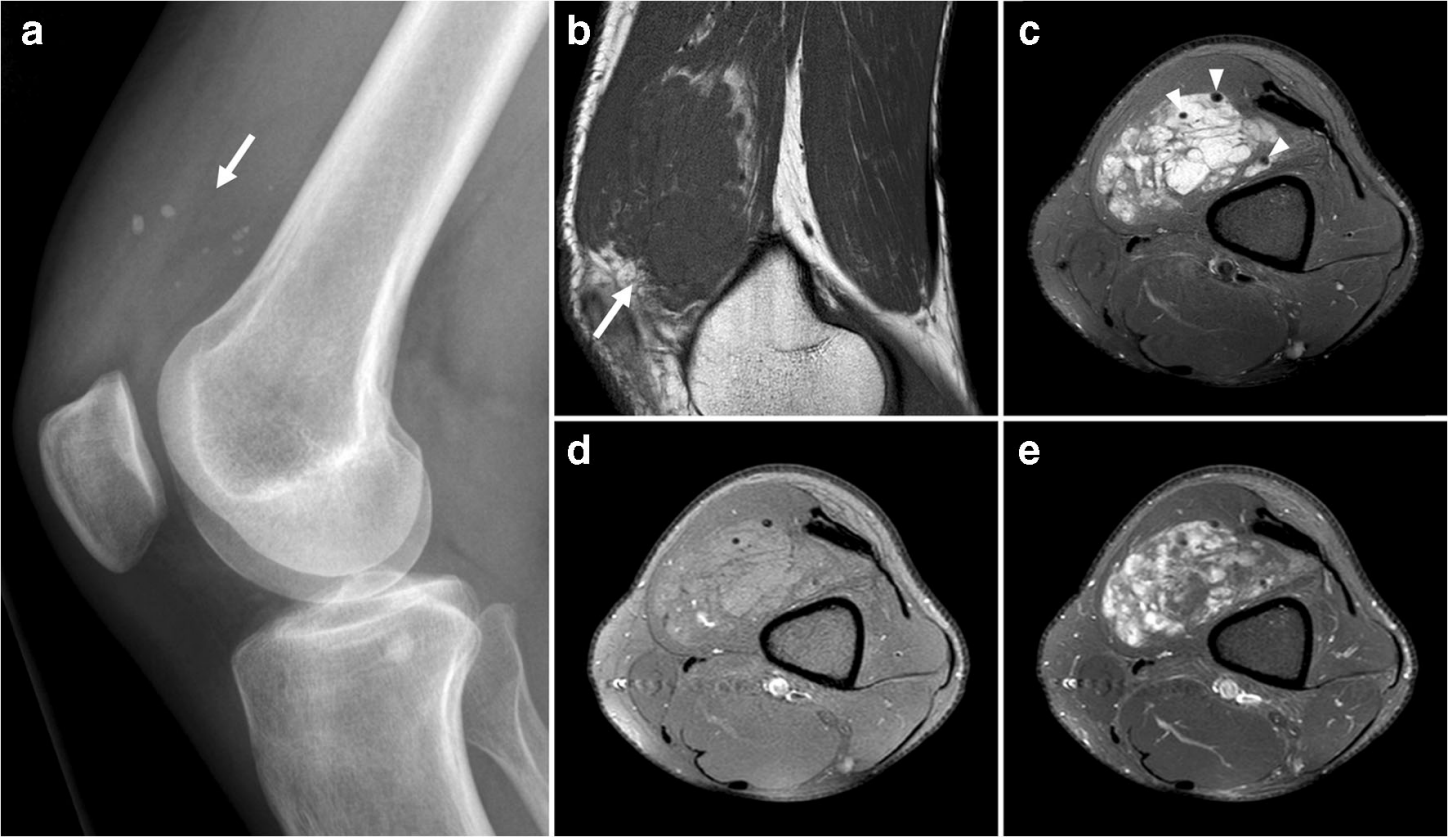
A 38-year-old man with a venous malformation. a Radiography shows suprapatellar phleboliths. b Sagittal T1-weighted image shows a lobulated mass with low signal intensity. c Axial proton-density weighted image with fat suppression shows the mass in the vastus medialis muscle to have high signal intensity and internal signal voids (*arrowheads*), representing phleboliths. d, e Axial pre- (d) and postgadolinium (e) T1-weighted images with fat suppression show enhancement of the mass. In this figure, fat overgrowth and serpentine morphology are also keys to the correct diagnosis of venous malformation
